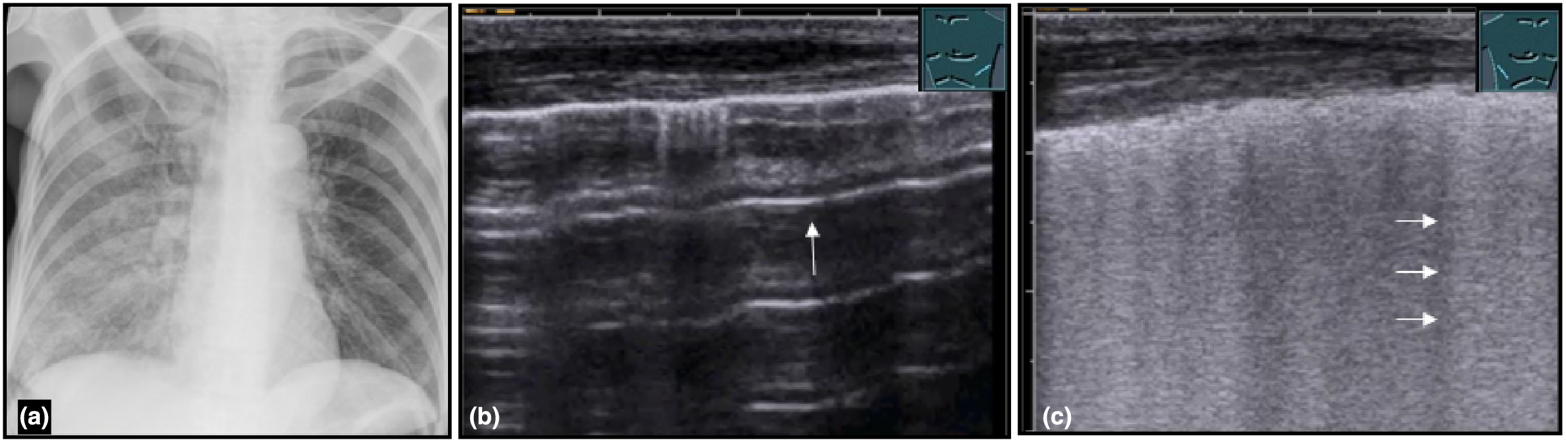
A 57‐year‐old female patient presented to the emergency department with weakness and a dry irritable cough. A chest X‐ray (courtesy of Prof. Dr. Andreas H. Mahnken, Department of Radiology, University Hospital Marburg) (a) shows a right‐sided infiltration of the lung; B‐mode ultrasound shows the healthy left lung (b), with a smooth, pleural entry echo and multiple A‐lines (arrow); and a right‐sided lung (c) with a reflexogenic lung with an irregular pleural entry echo and multiple B‐lines (arrows), consistent with community‐acquired interstitial pneumonia.
